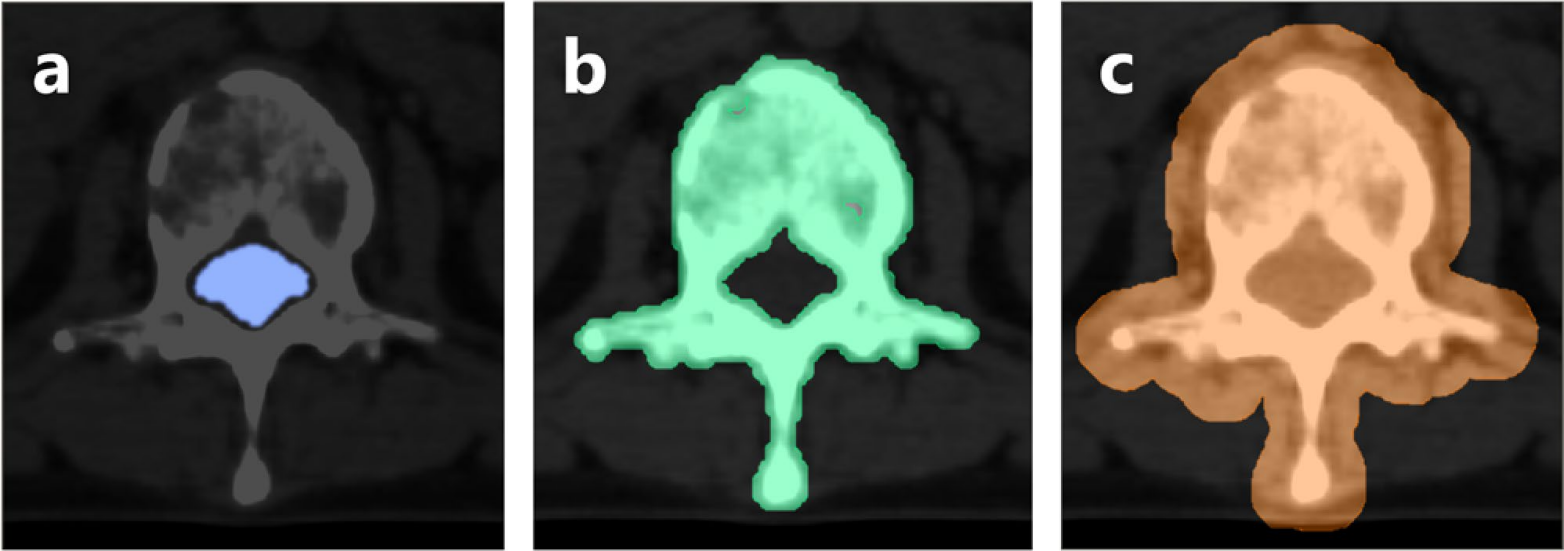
Segmentation of three regions of interest from CT images of patients with spinal metastases. (a) Spinal canal; (b) spine; (c) spine and surrounding tissues.
